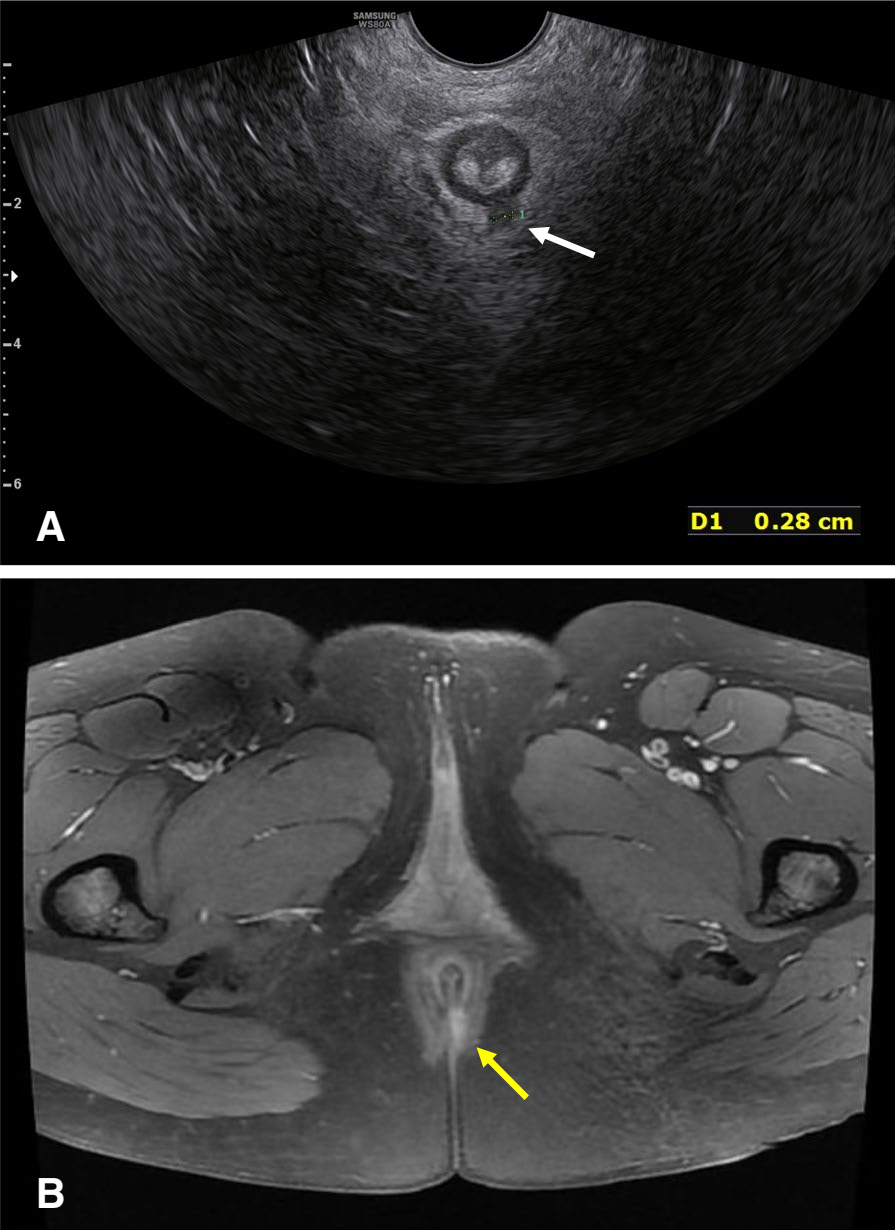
Anal fistula type II in pelvic 3D US and MRI images. A Pelvic 3D US image of a anal fistula(white arrow); (B)MRI image of a anal fistula(yellow arrow)
